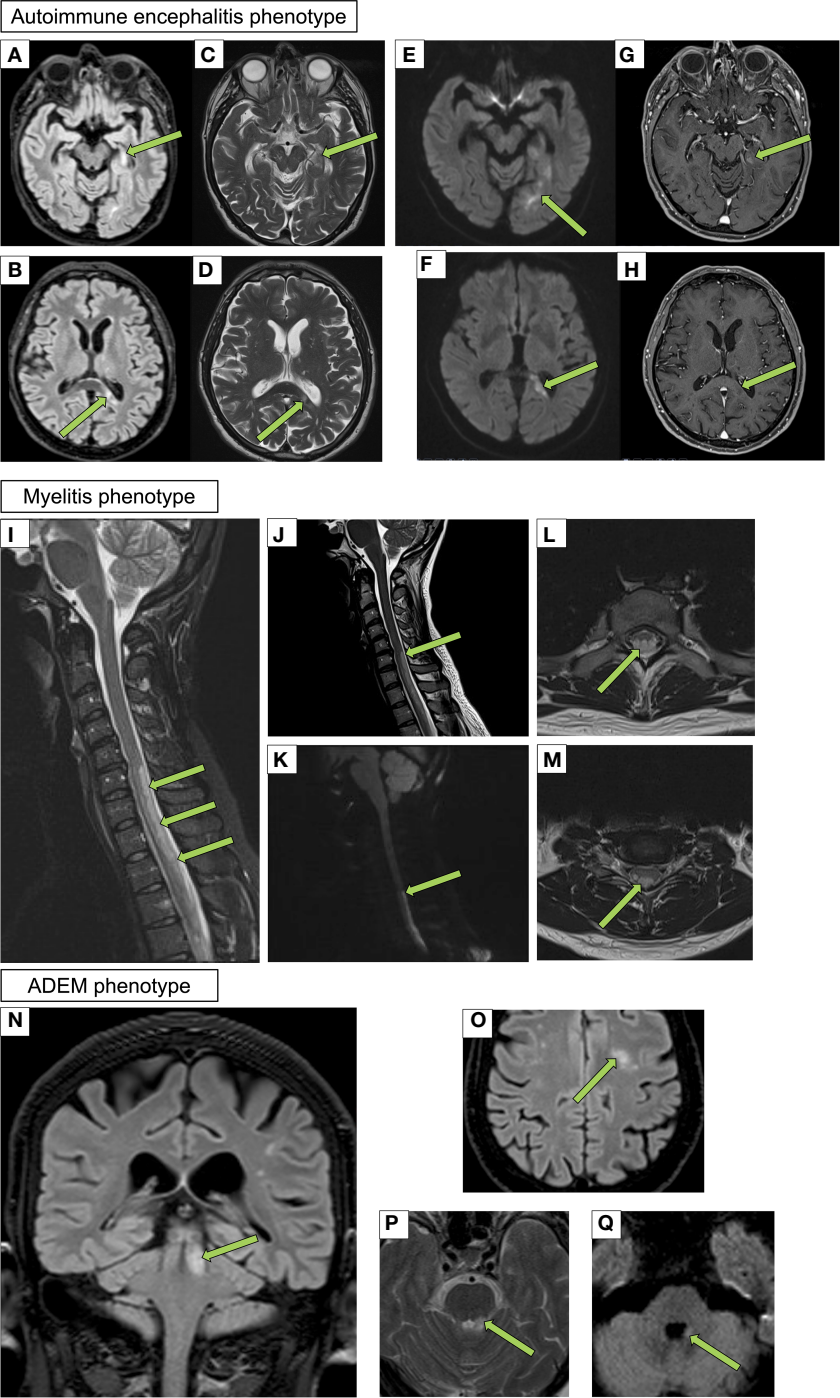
Neuroimaging findings in patients with CNS immune-mediated disorders after SARS-CoV-2 vaccination Autoimmune encephalitis phenotype (Case #7): axial fluid-attenuated inversion recovery (FLAIR) (A, B), turbo spin echo (TSE) T2 weighted (C, D), diffusion weighted (E, F) and Vibe T1 weighted after gadolinium administration (G, H) showing two different continuous lesions with restricted diffusion and patchy enhancement involving the left hippocampus and splenium of the corpus callosum. Myelitis phenotype (Case #4): sagittal short tau inversion recovery (STIR) (I), TSE T2 weighted (J), diffusion weighted (K) sequences and axial TSE T2 weighted (L, M) sequences of the cervical and upper thoracic spine showing a hyperintense cord lesion that extends over four contiguous vertebral segments. The lesion is characterized by restricted diffusion and involves the dorsal columns of the spinal cord. Acute disseminated encephalomyelitis (ADEM) phenotype (Case #2): coronal (N) and axial FLAIR (O), axial TSE T2 weighted (P) and diffusion weighted imaging (Q) sequences showing two different hyperintense lesions also with restricted diffusion (Q) involving the left superior cerebellar peduncle and white matter of centrum semiovale.
